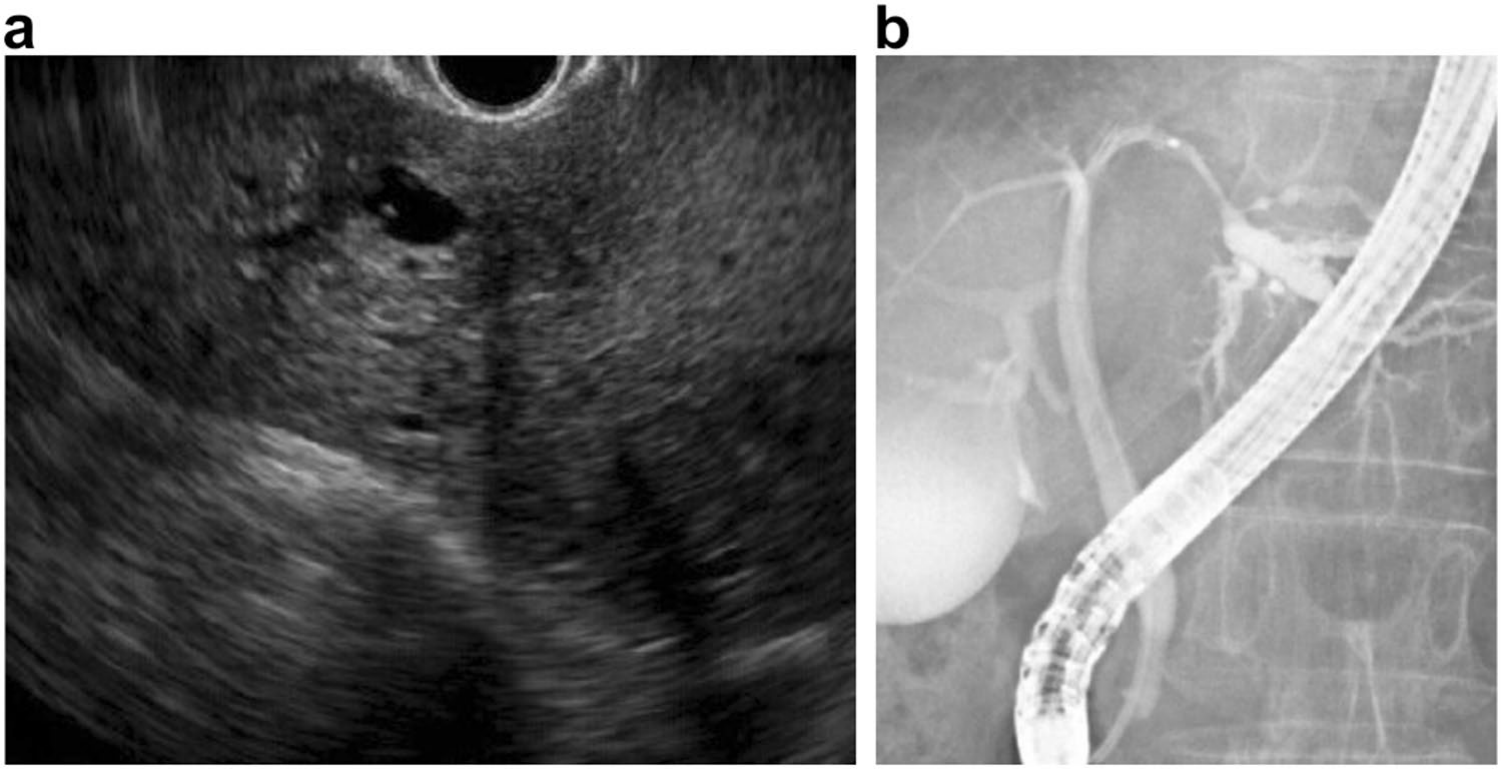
a Endoscopic ultrasound (EUS) image showing dilatation of B3 and a poorly marginated hypoechoic area. b Endoscopic retrograde cholangiopancreatography (ERCP) image showing smooth narrowing of the bile duct in B3 and a partially beaded appearance of B3
